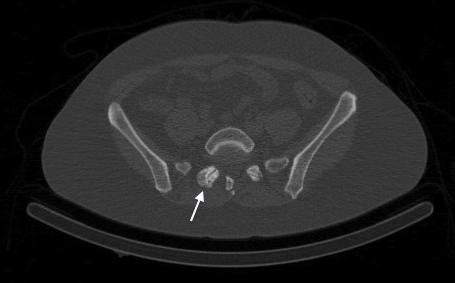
CT image from SPECT/CT demonstrating marked degenerative arthrosis at right L5/S1 facet (white arrow).
